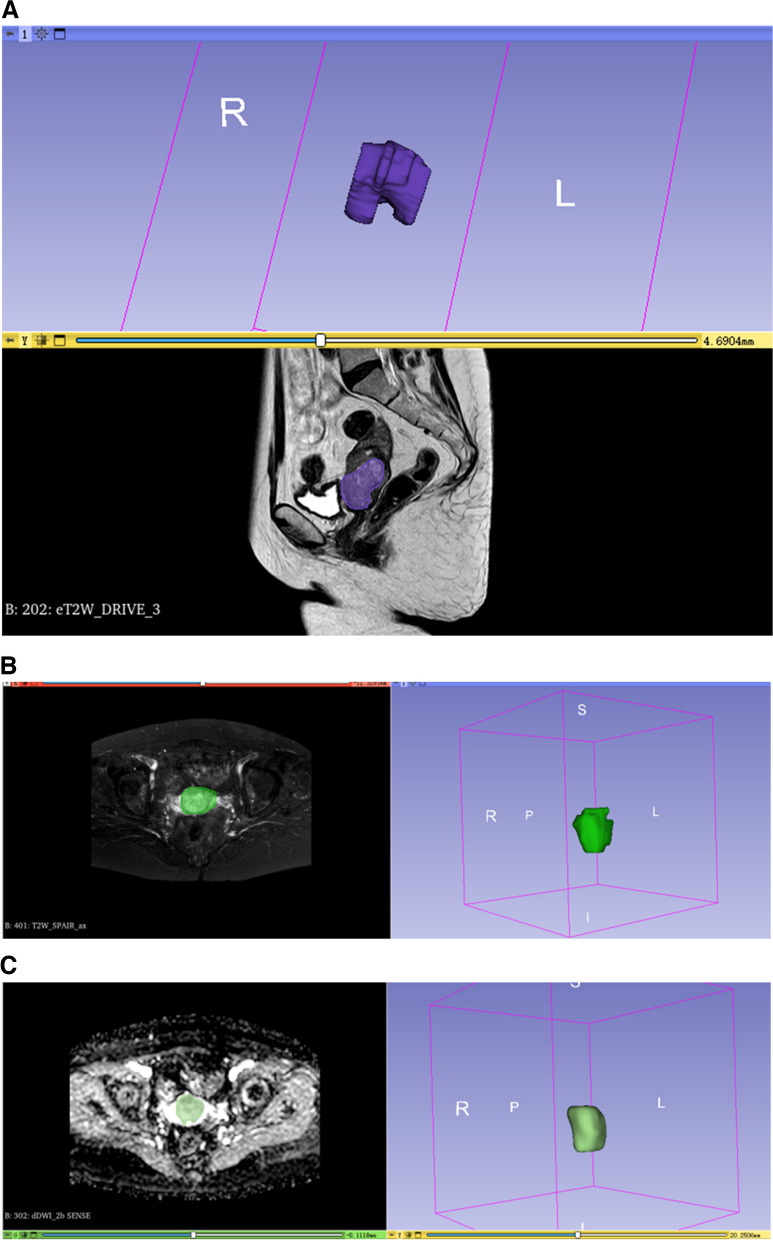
The figures of MRI segmentation on the sequences of a T2WI, b T2WI-SPAIR, and c ADC
